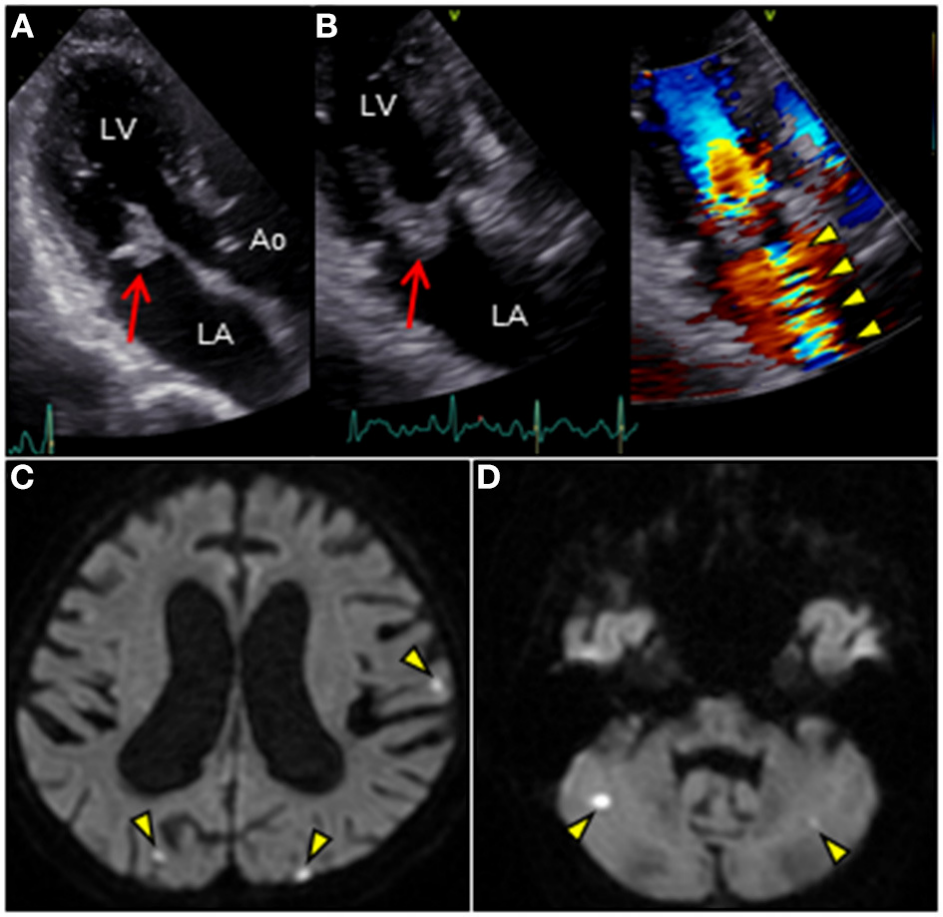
(A,B) Transthoracic echocardiography in apical three-chamber view reveals an irregular mass (18 × 17 mm, arrow) attached to the atrial side of the anterior mitral leaflet. Color Doppler image reveals massive mitral regurgitation traversing the mass (arowheads). (C,D) Brain diffusion-weighted magnetic resonance imaging reveals multiple acute hyperintense ischemic strokes in both hemispheres (arrowheads). Ao, aorta; LA, left atrium; LV, left ventricle.
